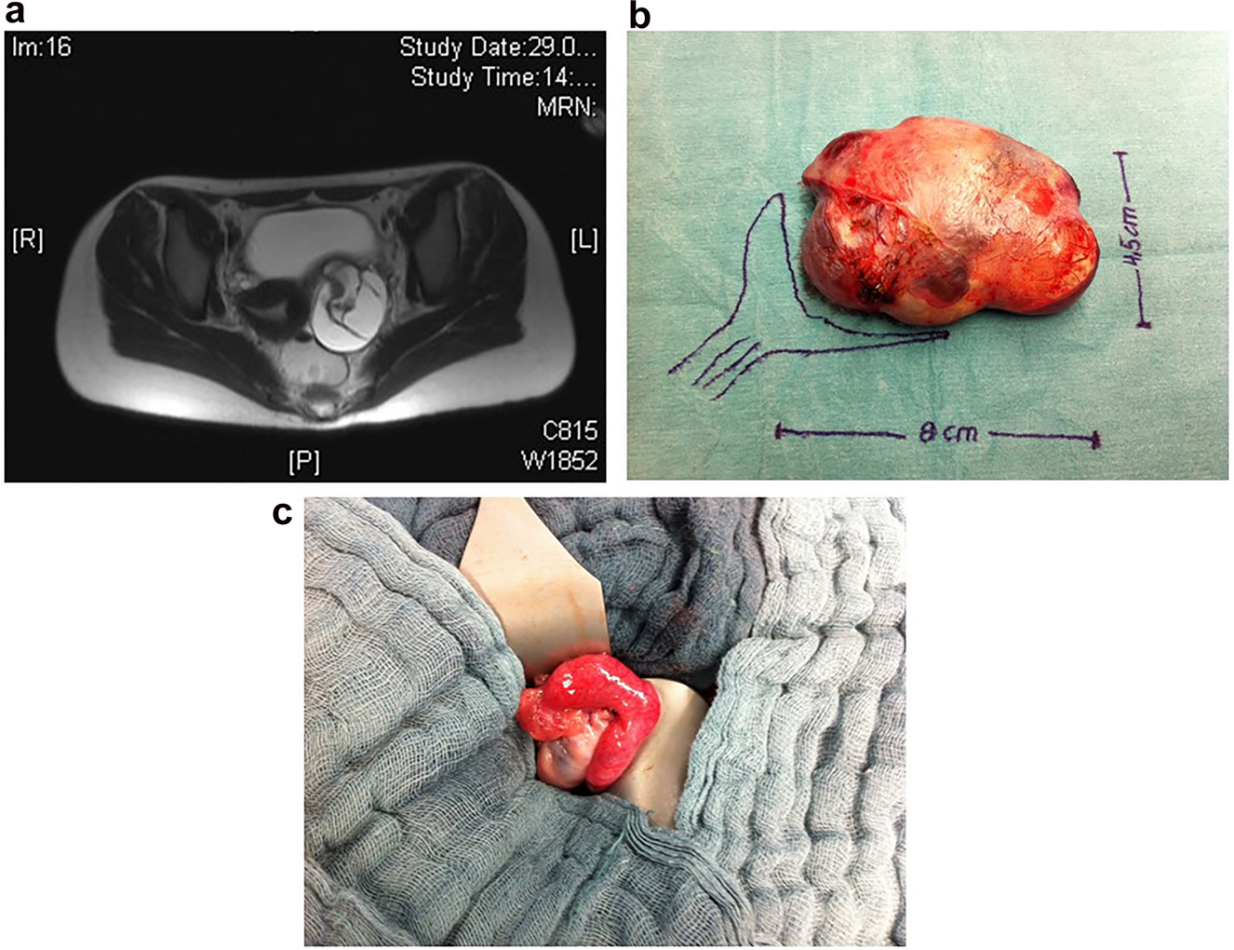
MRI scan (a) showed a big cystic-solid tumor of the ovary suggesting ovarian teratoma. AFP values were normal. *Ex-situ* photograph of the opened resected tumor showed the typical appearance of mucous and hairy contents of a mature teratoma (b). Intra-OP picture showed partial resection of the ovarian mass, preserving healthy ovarian tissue (c).
